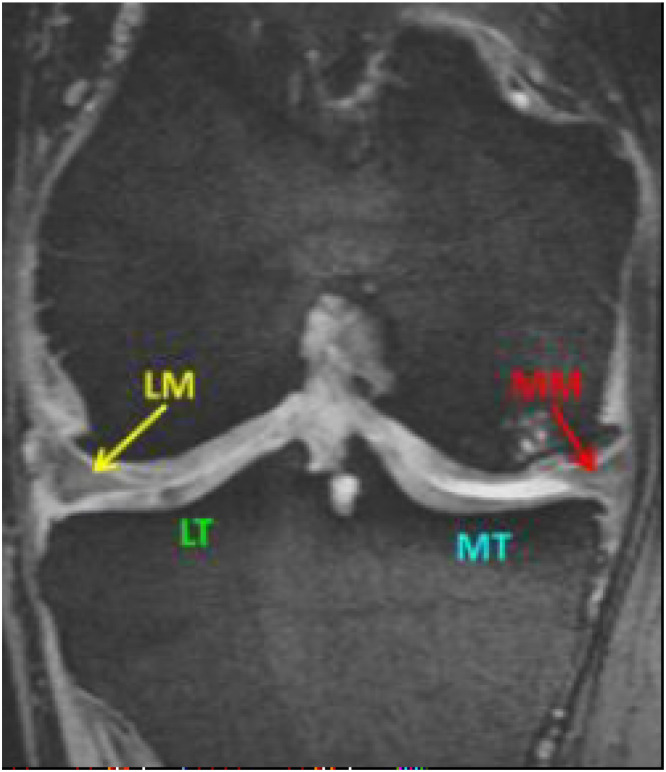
MRI showing the medial meniscus (MM), lateral meniscus (LM) and the cartilage surface areas as medial tibia (MT) and the lateral tibia (LT).
